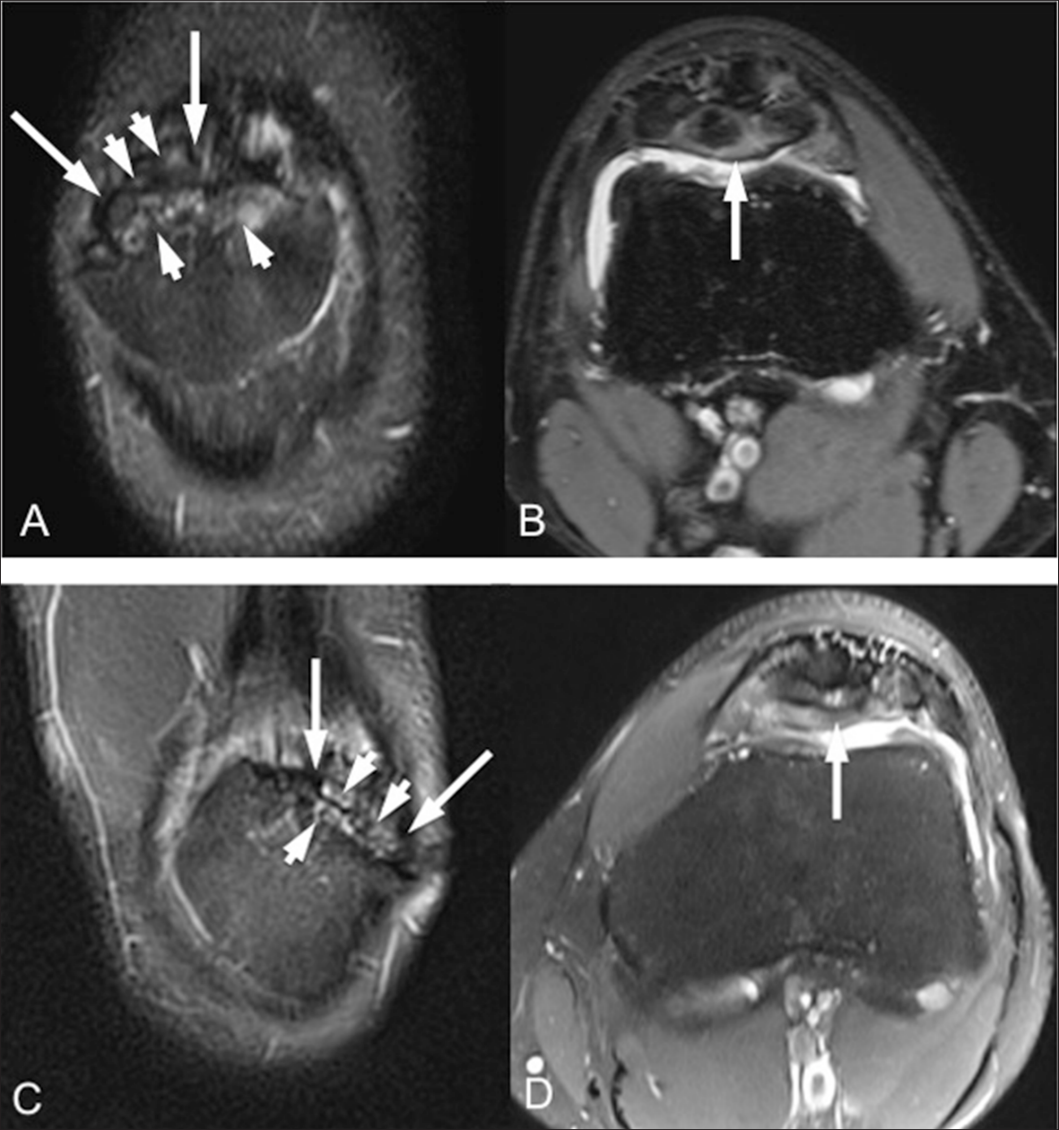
Coronal fat-saturated T2-weighted (A, C) and axial fat-saturated proton-density-weighted (B, D) MR images (respectively for right and left knee) show bilateral multipartite patella variation (long arrows) with bone marrow edema-like signal changes within the bony fragments (short arrows).
